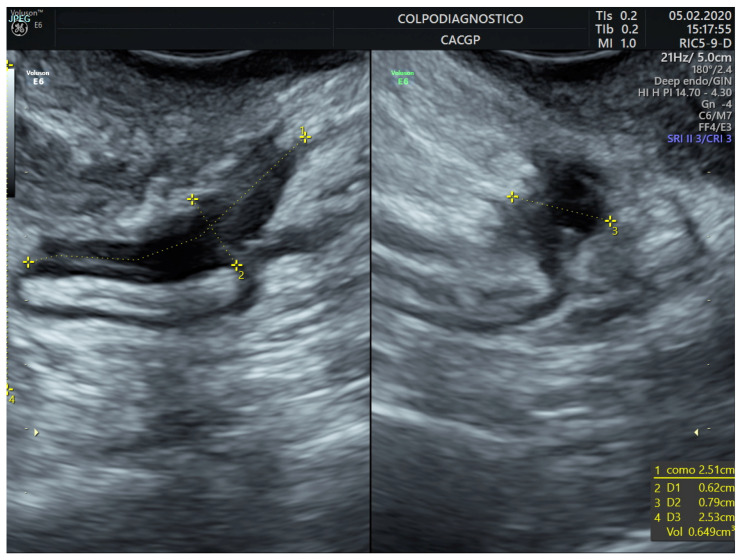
Transvaginal ultrasound image showing the measurements performed in an endometriotic nodule in the sigmoid colon.
